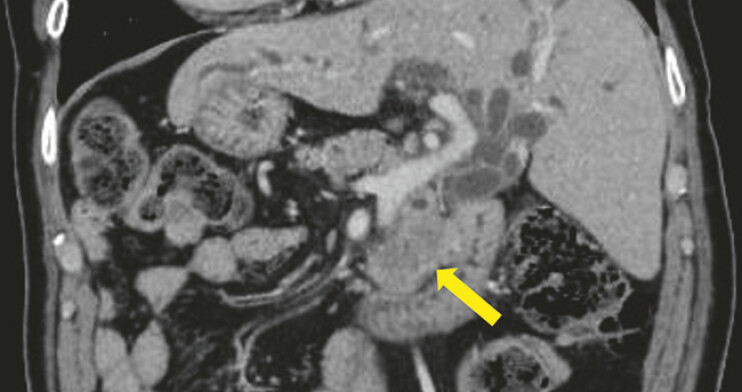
Computed tomography (CT) demonstrating situs inversus totalis (SIT) and pancreatic cancer (yellow arrow).
